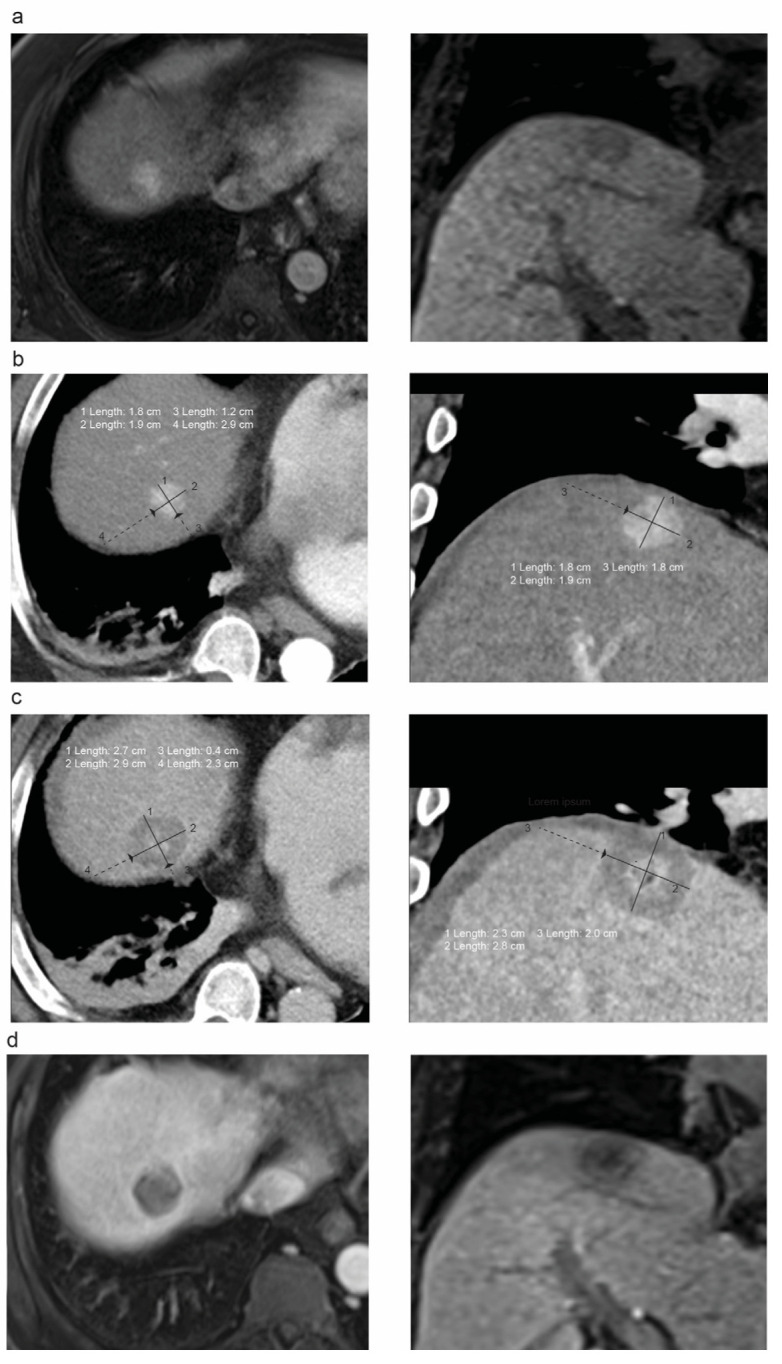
Example of minimal ablative margin assessment in MRI/CT scans of a 67-year-old man with HCC: (a) hepatic contrast-enhanced MRI (left: arterial phase, right: hepatobiliary phase) before MWA treatment; (b) contrast-enhanced planning CT scan (arterial phase) with pre-interventional measurements as performed in our study; (c) contrast-enhanced control CT scan (portal venous phase) after complete tumor ablation with postinterventional measurements; (d) contrast-enhanced follow-up MRI (left: arterial phase; right: hepatobiliary phase) 6 months after MWA with no evidence for local tumor progression.
